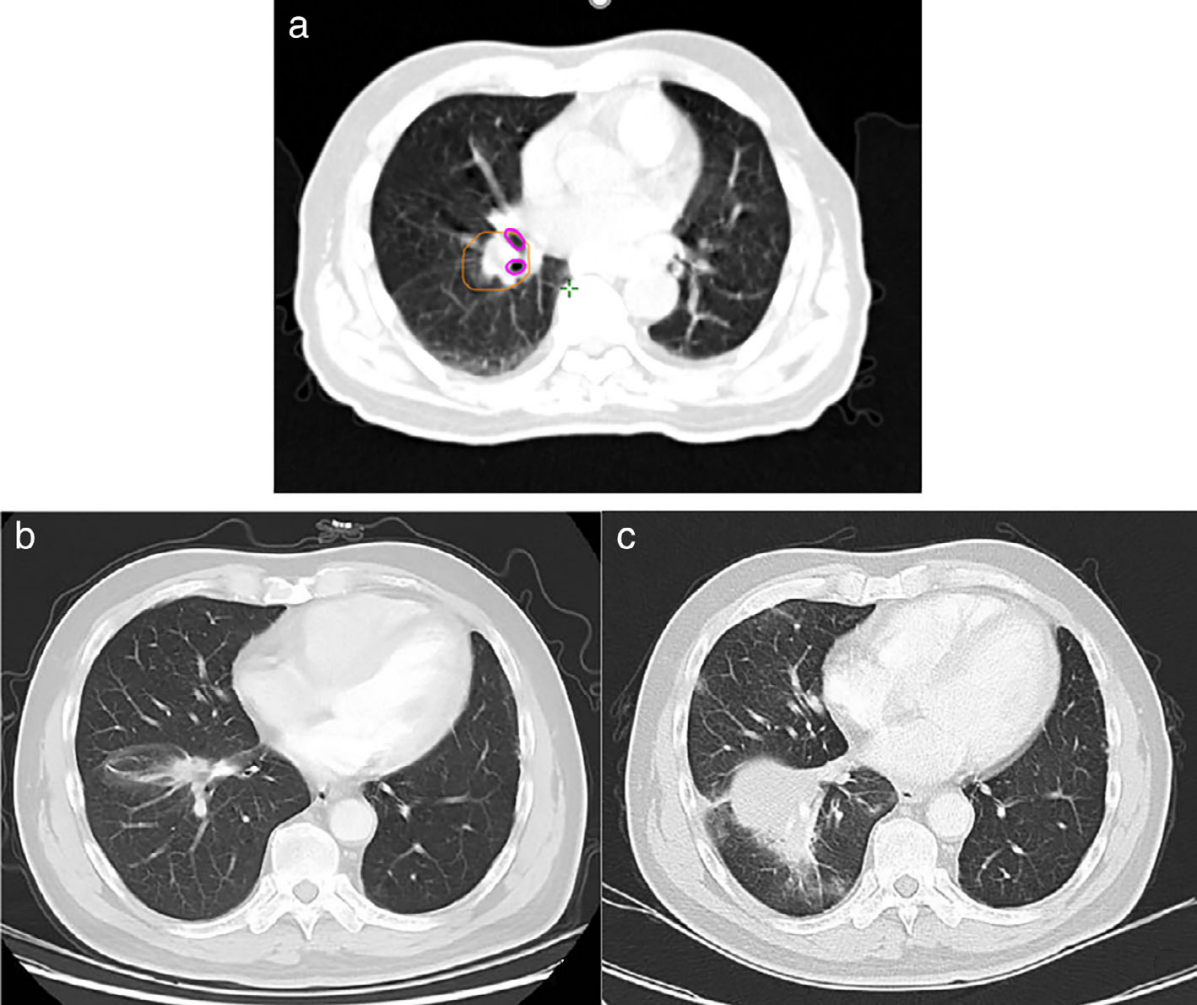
Radiotherapy plan and chest CT of the patient with grade 1 atelectasis. (a) Shows the radiotherapy plan (planning target volume in orange, right middle lobar bronchus and right inferior lobar bronchus in purple). (b) Chest CT before SBRT. (c) Chest CT after SBRT, which is in the same slice as (b) and shows grade 1 atelectasis.
